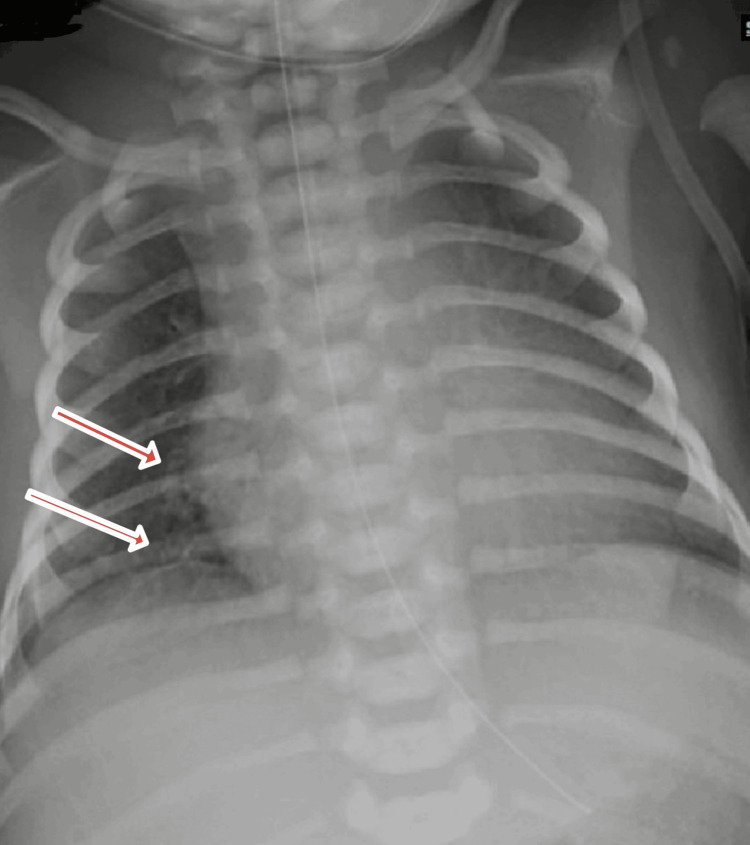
Chest X-ray on day 1 of life showing mild bronchovascular prominence (arrows)
